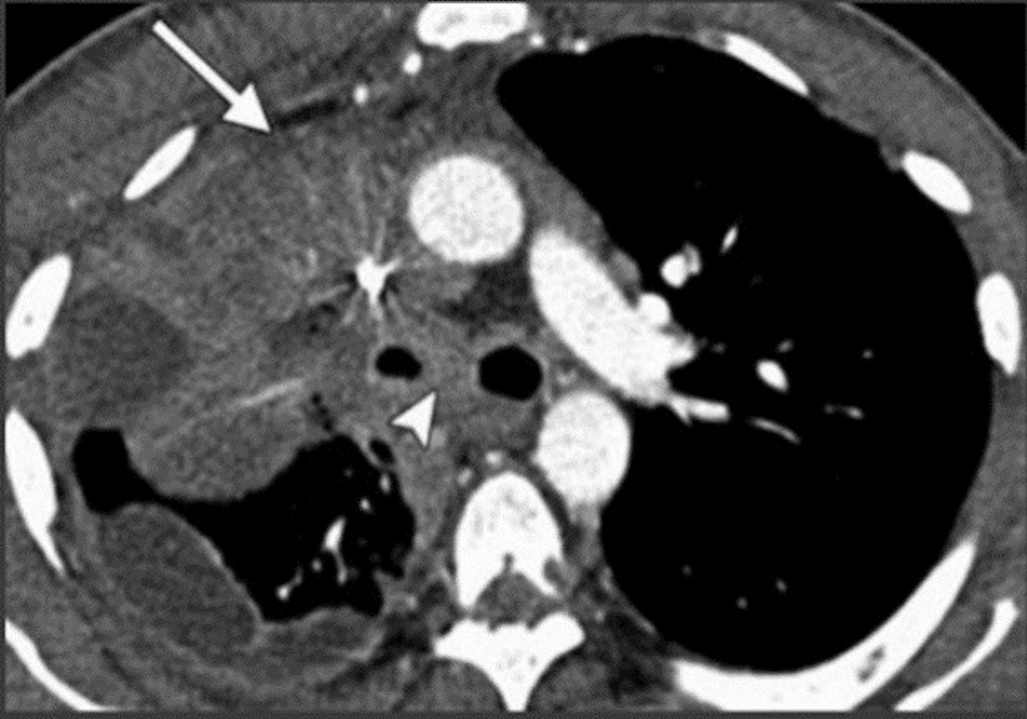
Computed tomography (CT) chest scan. CT chest shows a right upper lobe mass with mediastinal and carinal invasion, ipsilateral loculated pleural effusion, and thickening of the pleura.
